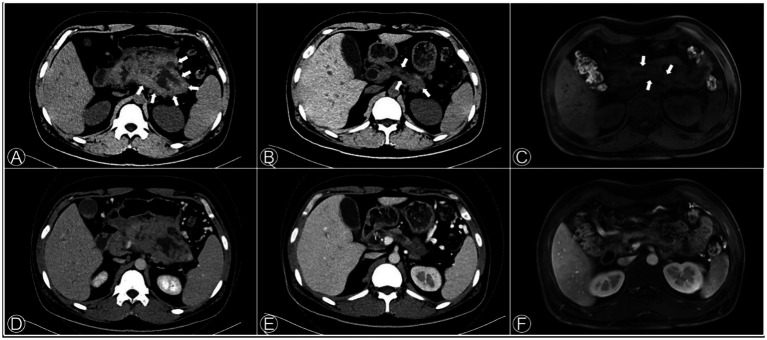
Imaging of infiltration of the pancreas and acute pancreatitis. (A–C) Non-contrast images. (D–F) Corresponding portal venous phase contrast-enhanced images at identical scan levels (A–D, B–E, C–F). (A,D) Abdominal computed tomography (CT) showed pancreatic necrosis with diffuse peripancreatic inflammatory exudation (white arrow). (B,E) Abdominal contrast-enhanced CT showed a reduction in pancreatic size compared with prior imaging, along with decreased peripancreatic inflammatory exudation (white arrow). (C,F) Upper abdominal magnetic resonance imaging (MRI) showed a reduction in pancreatic enlargement and decreased peripancreatic inflammatory exudation compared with the previous CT examination (white arrow).
